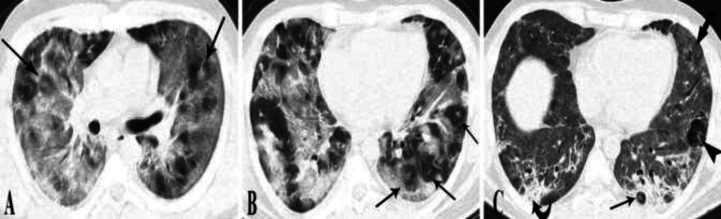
Serial imaging of case 2. The on-admission chest CT scan (A-B) depicts diffuse GGO and small foci of poorly developed target appearances (arrows). The second CT scan five days later shows diffuse residual faint GGO (thick arrow), parenchymal subpleural bands (curved arrow), and consolidating changes in both lower lobes. A few bullae (arrowhead) are also developed in the periphery of almost all pulmonary lobes. A small focus of target appearance is also present (arrow)
